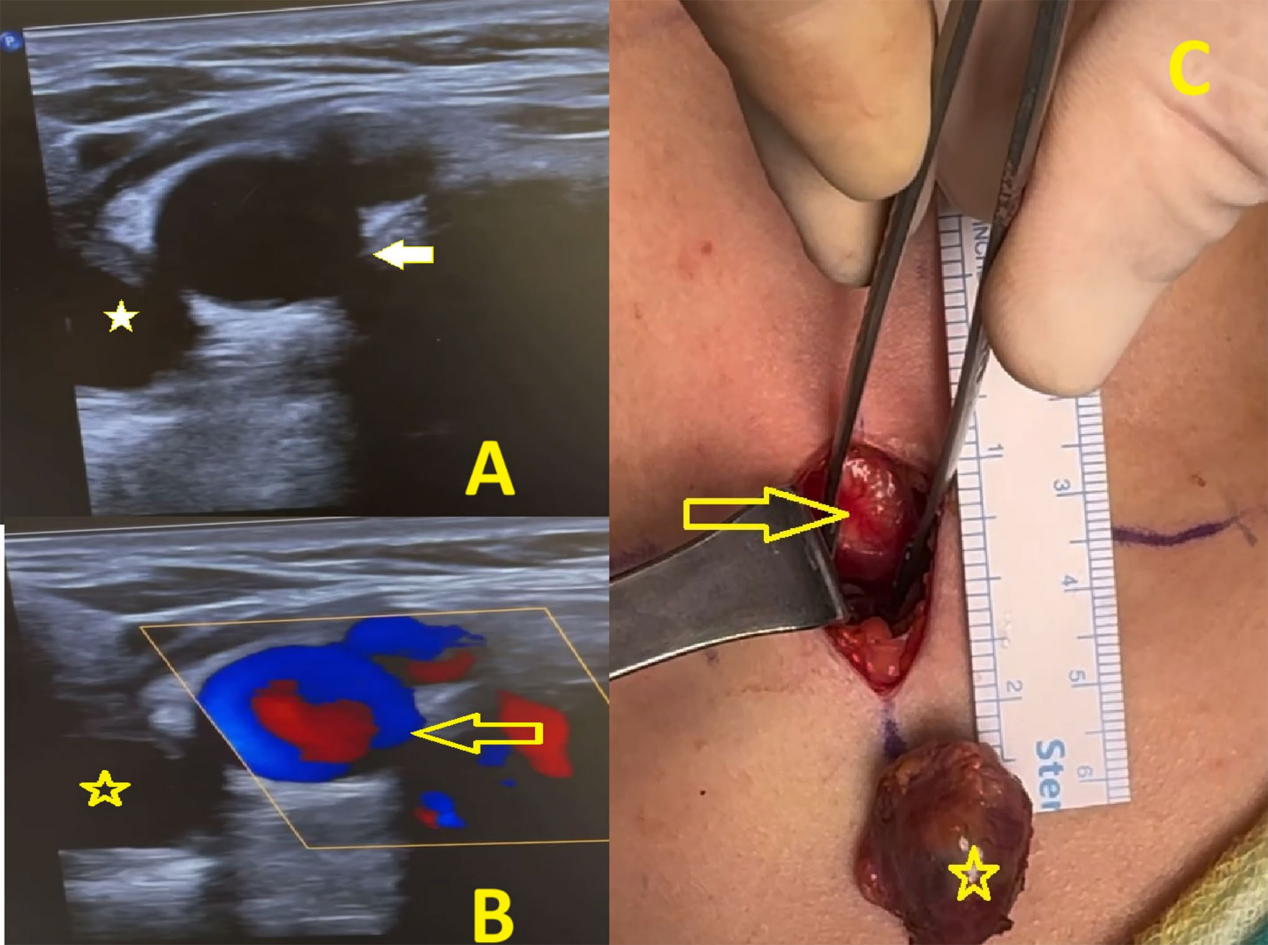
An ectopic parathyroid adenoma. A: Neck ultrasonography (surgeon-performed) sagittal section, white arrow right brachiocephalic- common carotid arteria * right inferior ectopic parathyroid adenoma. B: Neck doppler ultrasonography (surgeon-performed) sagittal section, yellow arrow right brachiocephalic common carotid arteria, * right inferior ectopic parathyroid adenoma. C: Intraoperative görüntü, yellow arrow brachiocephalic arteria, * parathyroid adenoma
